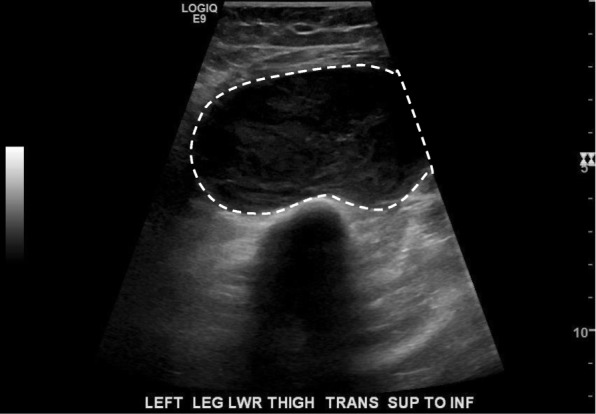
Ultrasound imaging of left thigh mass. Mass outlined with *dashed white line*
